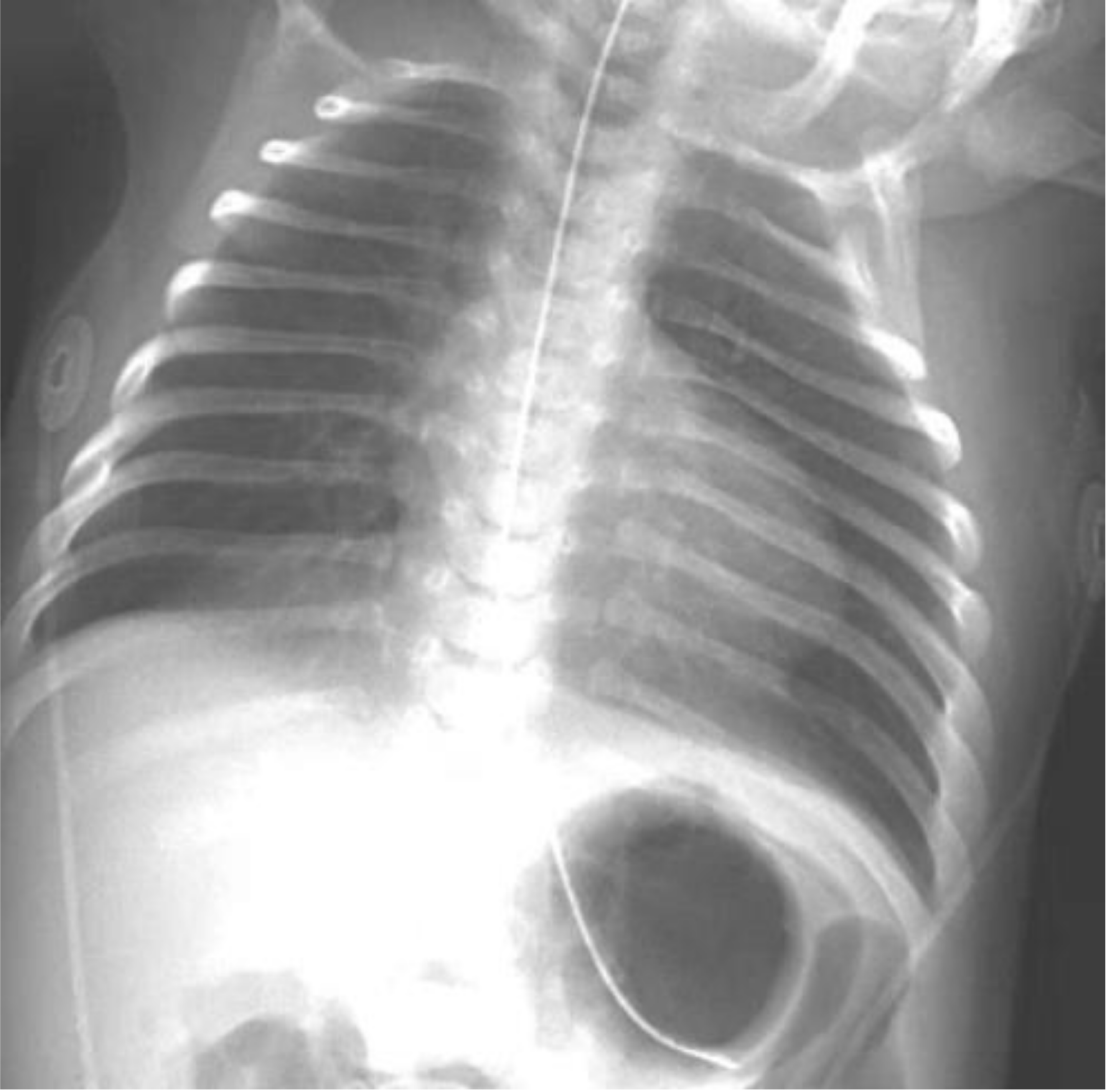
Frontal chest radiograph of an infant showing ribs, vertebral column, diaphragm, and part of the abdomen, with a nasogastric tube extending down the midline toward the stomach region. Thymic shadow is not evident.
